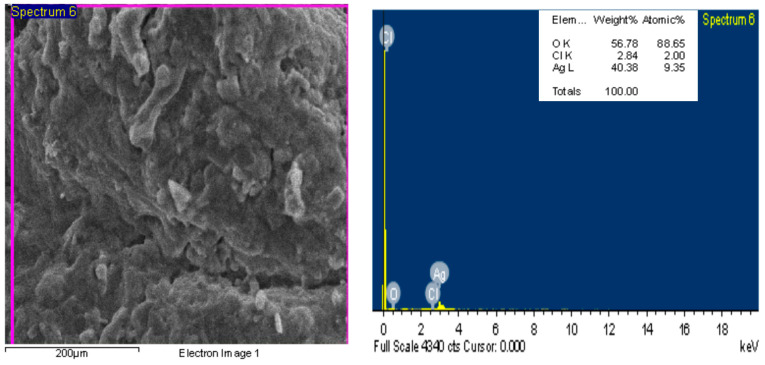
Energy-dispersive X-ray (EDX) of Tt-AgNPS derived from marine alga *T. turbinata*.
